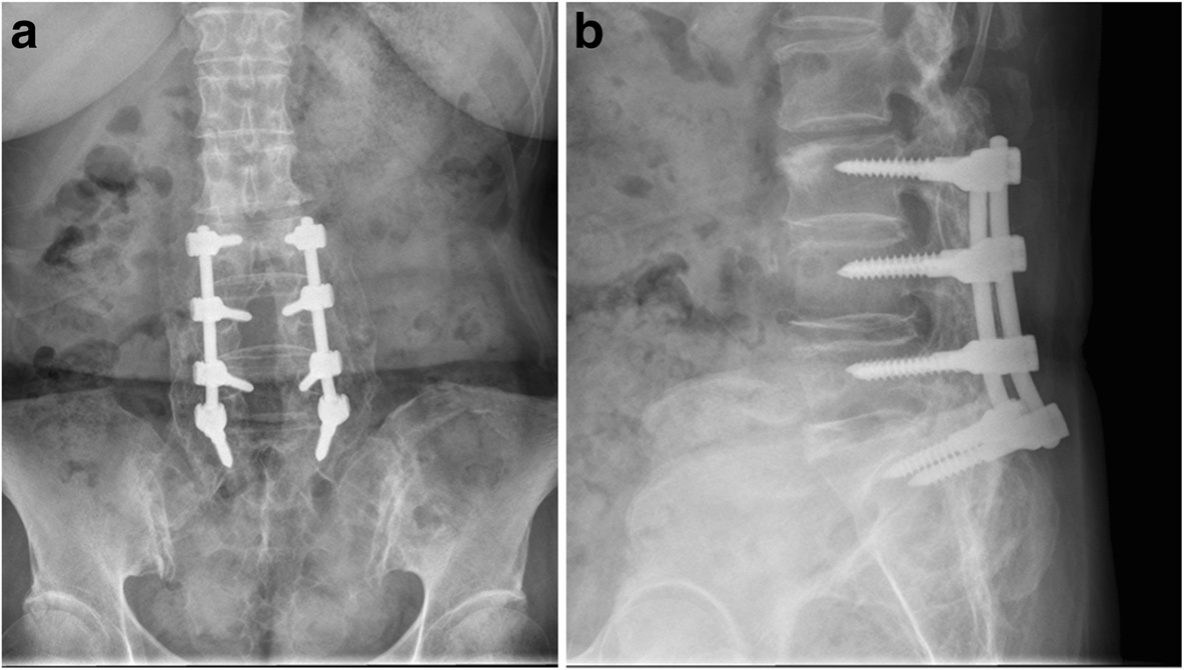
Post-fusion radiographs of a 67-year-old man showing a good fusion mass at 12 years and 1 month of follow up. a anteroposterior view. b lateral view
